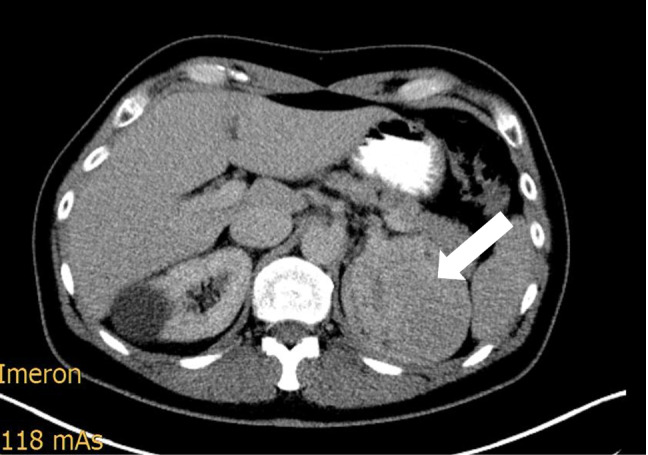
Exemplary CT scan of a female patient with a 9.5 cm left cortisol-producing adrenal adenoma
